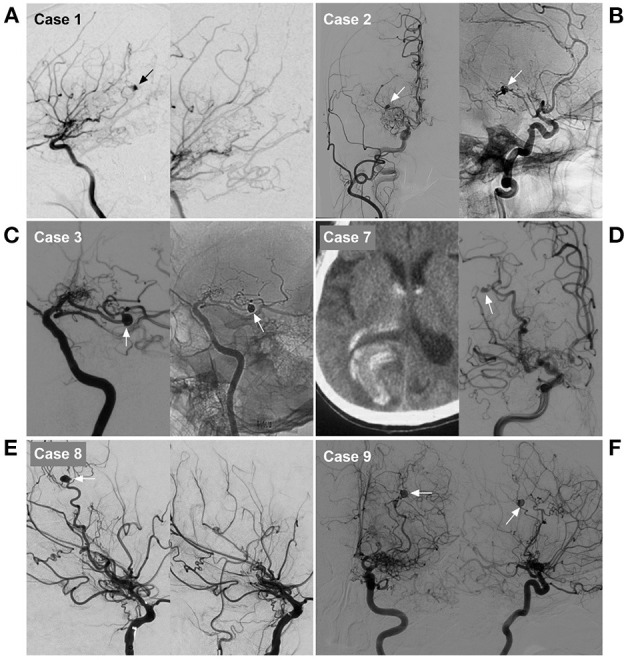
Imaging characteristics of cases 1, 2, 3, 7, 8 and 9 in Table 1. (A) Case 1: Left, DSA of the ICA showed an aneurysm on the distal AchA (arrow). Right: Postoperative DSA showing that both the parent artery and aneurysm were occluded with Onyx. (B) Case 2: Left, DSA of the carotid artery showed an aneurysm on the distal AchA (arrow). Right: Postoperative DSA showing that both the parent artery and aneurysm were occluded with coiling (arrow). (C) Case 3: Left, DSA of the ICA showed an aneurysm on the distal AchA (arrow). Right: Postoperative DSA showing that both the parent artery and aneurysm were occluded with coiling (arrow). (D) Case 7: Left, CT showed hemorrhage of the right occipital lobe into the lateral ventricle. Right: DSA of the ICA showing the aneurysm (arrow) located on the P4–5 segment of the PCA. (E) Case 8: Left, DSA of the ICA showed an aneurysm on the distal LSA (arrow). Right: Postoperative DSA showing that both the parent artery and aneurysm were occluded. (F) Case 9: DSA of the ICA in the anterior-posterior view (left) and lateral view (right) showed an aneurysm on the distal LSA (arrow). AchA, anterior choroidal artery; CT, computed tomography; DSA, digital subtracted angiography; ICA, internal carotid artery; LSA, lenticulostriate artery; PAO, parent artery occlusion; PCA, posterior cerebral artery.
